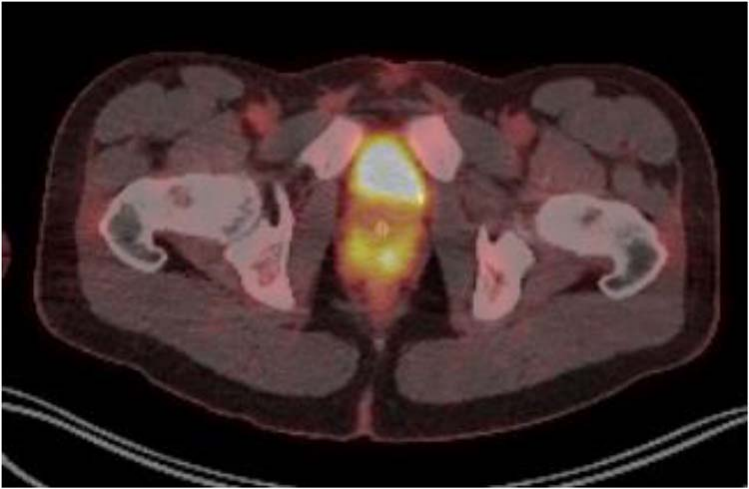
PET FDG scan pre-R-CHOP chemotherapy showing disease activity in the prostate.
